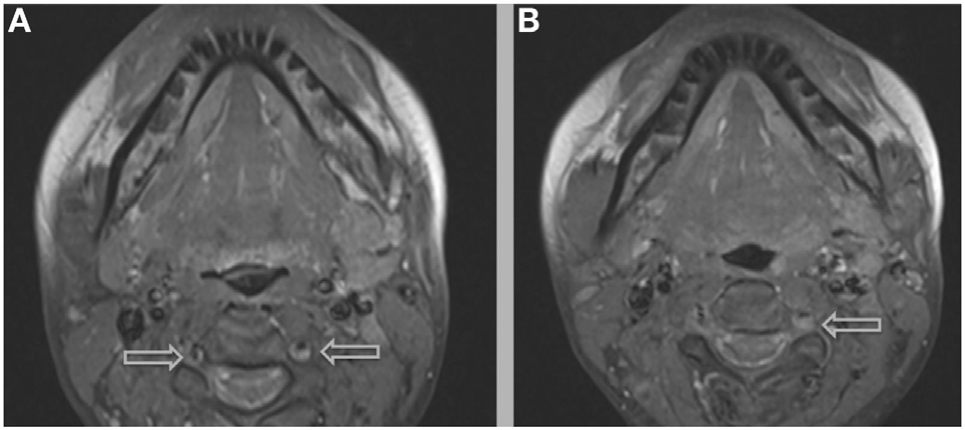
Additional MRI imaging indicating mural hematomas within the right (A,B) and left (B) vertebral arteries, findings that would resolve following anticoagulant therapy and the cessation of lenvatinib.
